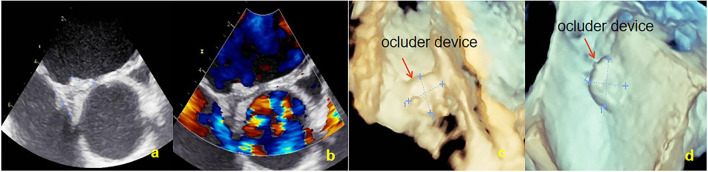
Overall outline of the device was observed by TEE at 12 months; both discs were approximately circular and completely fitted the atrial septum. (a) Two-dimensional TEE displaying the shape of NBO. (b) Doppler color flow imaging showing that there was no shunt in the atrial interval. (c) Three-dimensional TEE displaying the outline of the right side occluder. (d) Three-dimensional TEE displaying the outline of the left side occluder. TEE, Transesophageal echocardiography; NBO, Novel biodegradable occluder.
